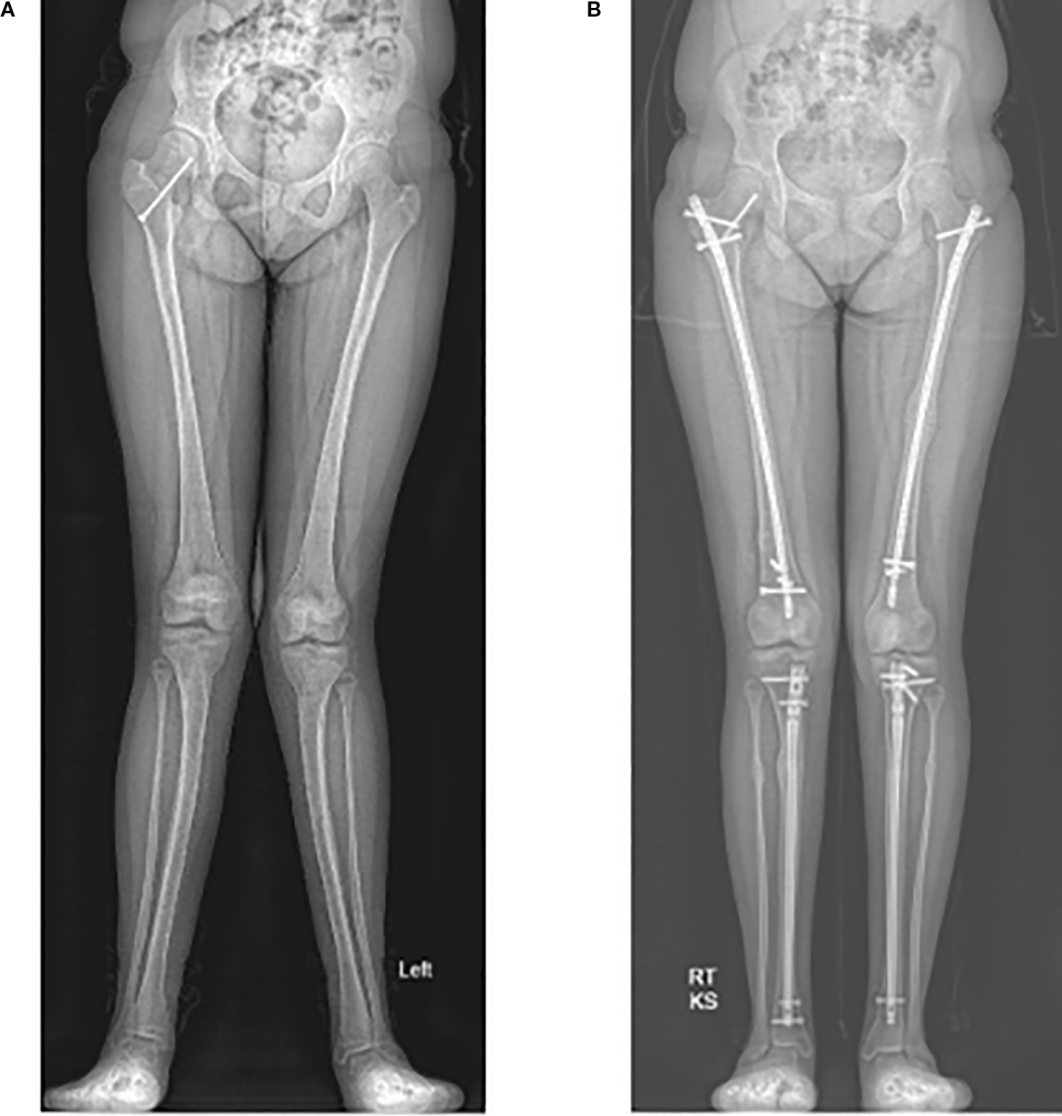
Radiographs of the same patient, who at 17 years of age underwent posterior spine fusion for thoracic-level scoliosis (A, B). Of note, he received a renal transplant at age 16.
